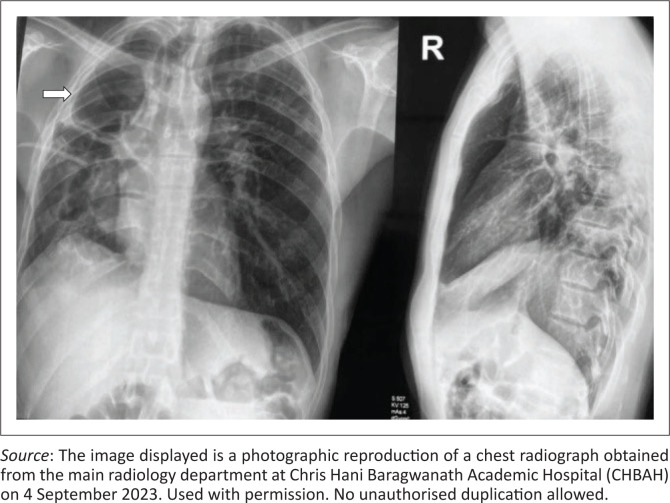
Chest radiographs (frontal and lateral projections) depicting right upper lobe fibrocavitary changes (white arrow) with ipsilateral tracheal deviation.
